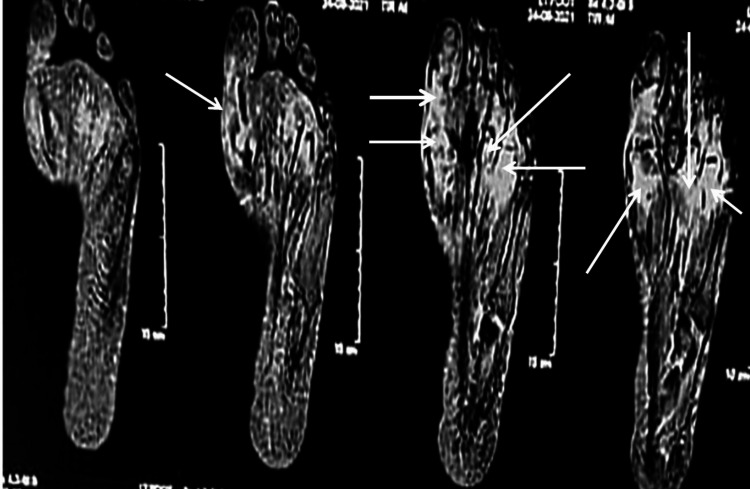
MRI shows ill-defined patchy marrow edema and associated soft-tissue edema of the head of the first, third, and fourth metatarsal bones, as well as edema of the shaft of the proximal phalanx of the fourth toe
